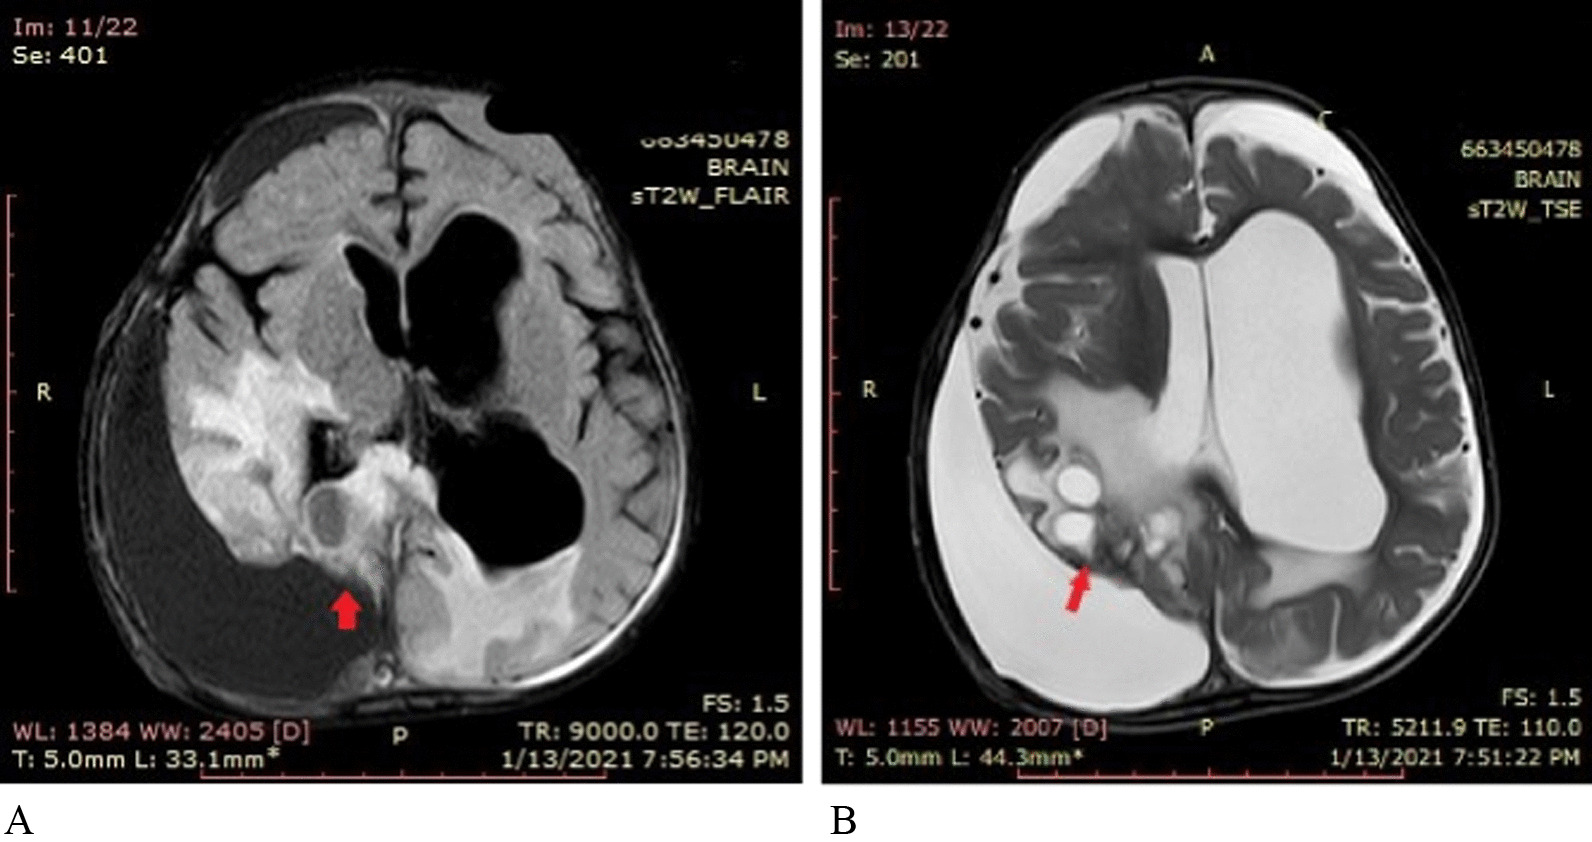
Two months postoperative MRI without contrast. A T1 images show an iso to hypo mass, B T2/FLAIR images show a mixed intensity image in a right occipital location adherent to the dura with moderate subdural collection
